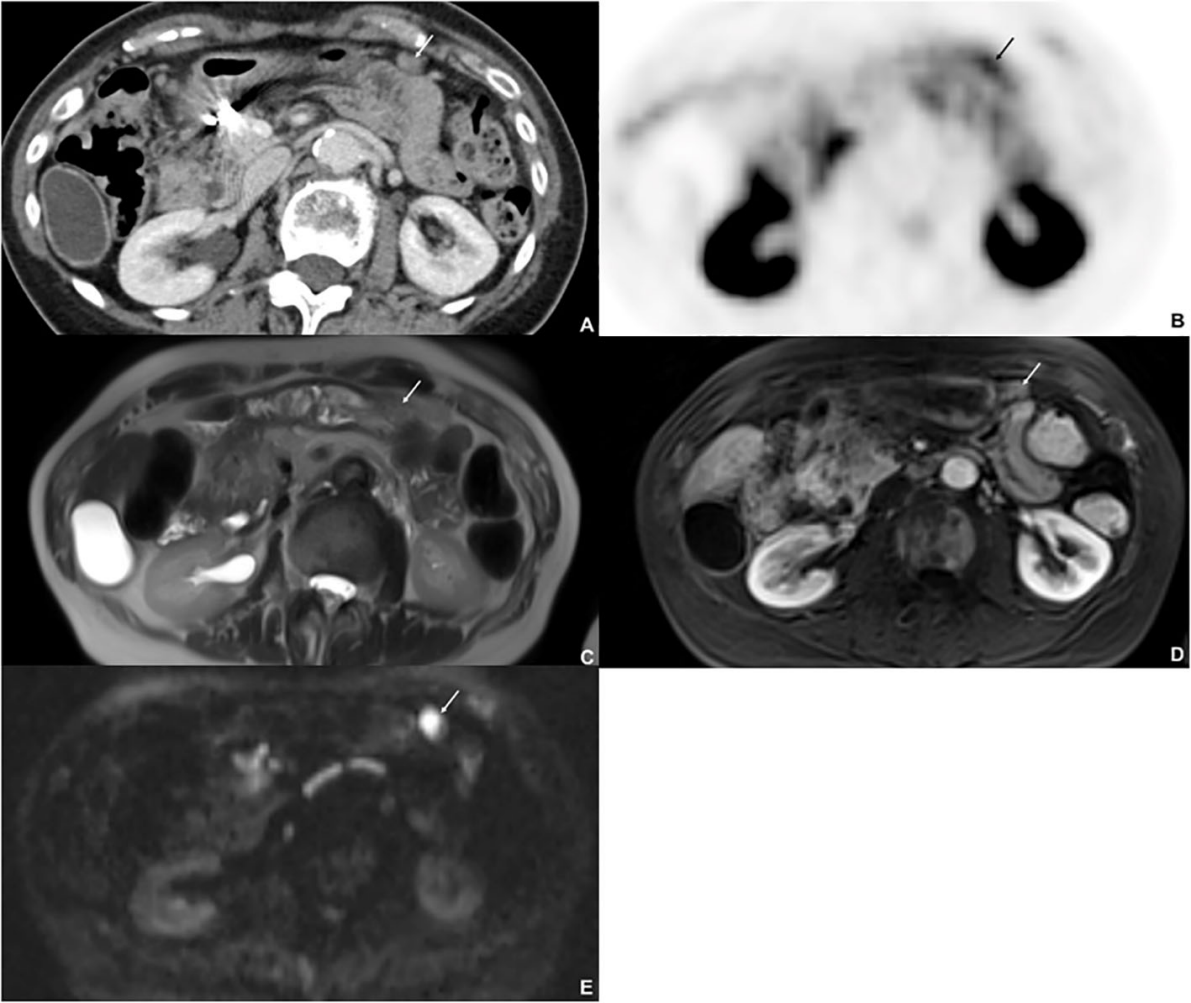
Seventy-seven-year-old female with metastatic NET, originating from the lung. ^68^Ga-DOTATATE PET/CT shows a peritoneal lesion on CT (A) (arrow) at the anterior peritoneal surface with high uptake (B). The lesion was missed on T2w image (C) and on postcontrast T1w image (arterial phase) (D) but clearly detected on DWI (E): The lesion shows restricted diffusion.
